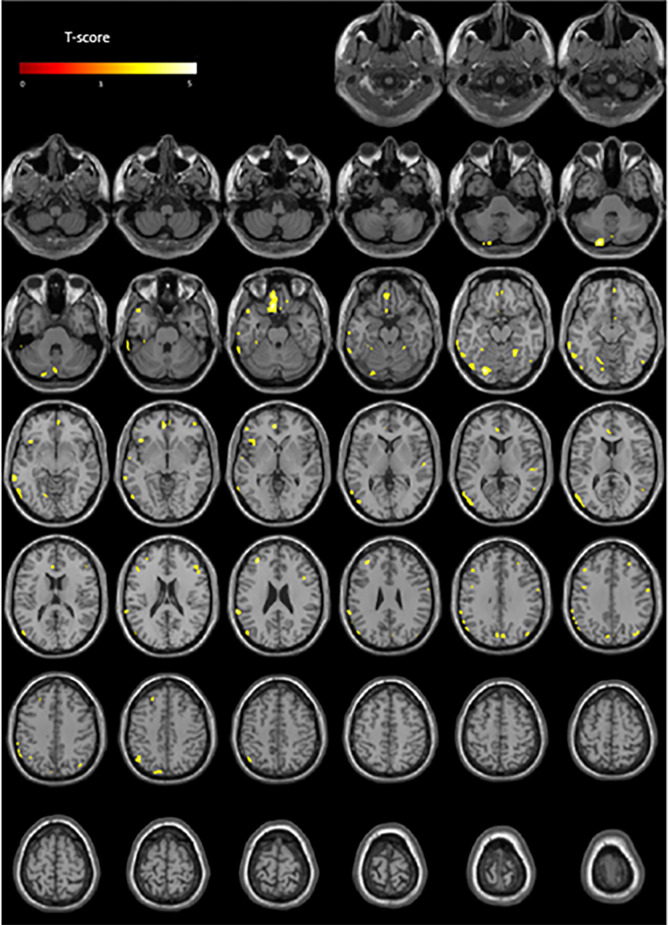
Anatomical localizations of significant brain metabolic clusters associated with HR (negative association) in the whole population (*p* < 0.001, uncorrected) projected onto two-dimensional slices of T1-weighted MRIs (from the base to the top of the skull).
